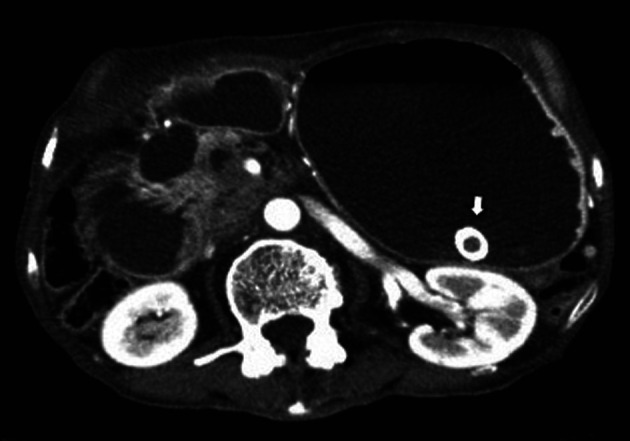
Abdominal CT showed the presence of the SEMS in the stomach (arrow).
